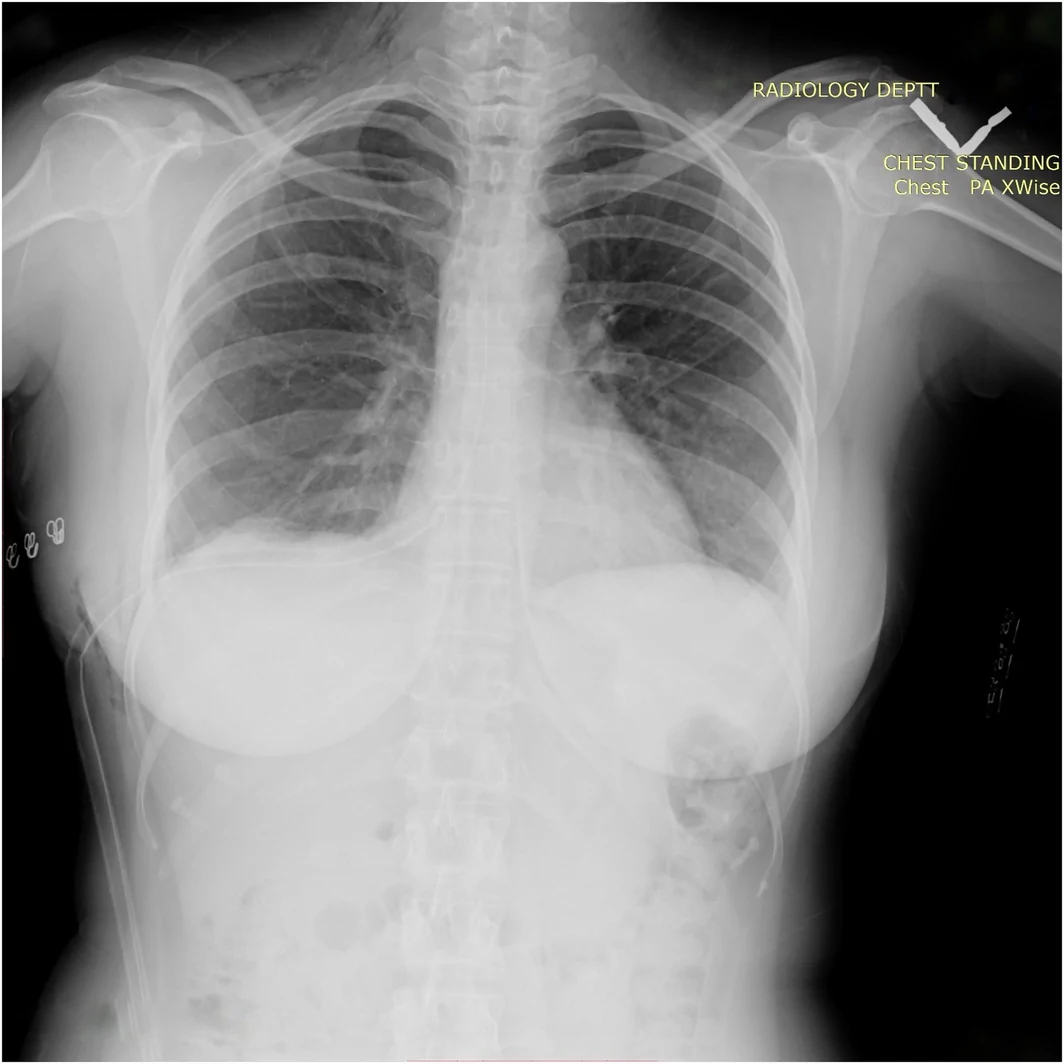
Chest radiograph after right‐sided chest tube insertion.
